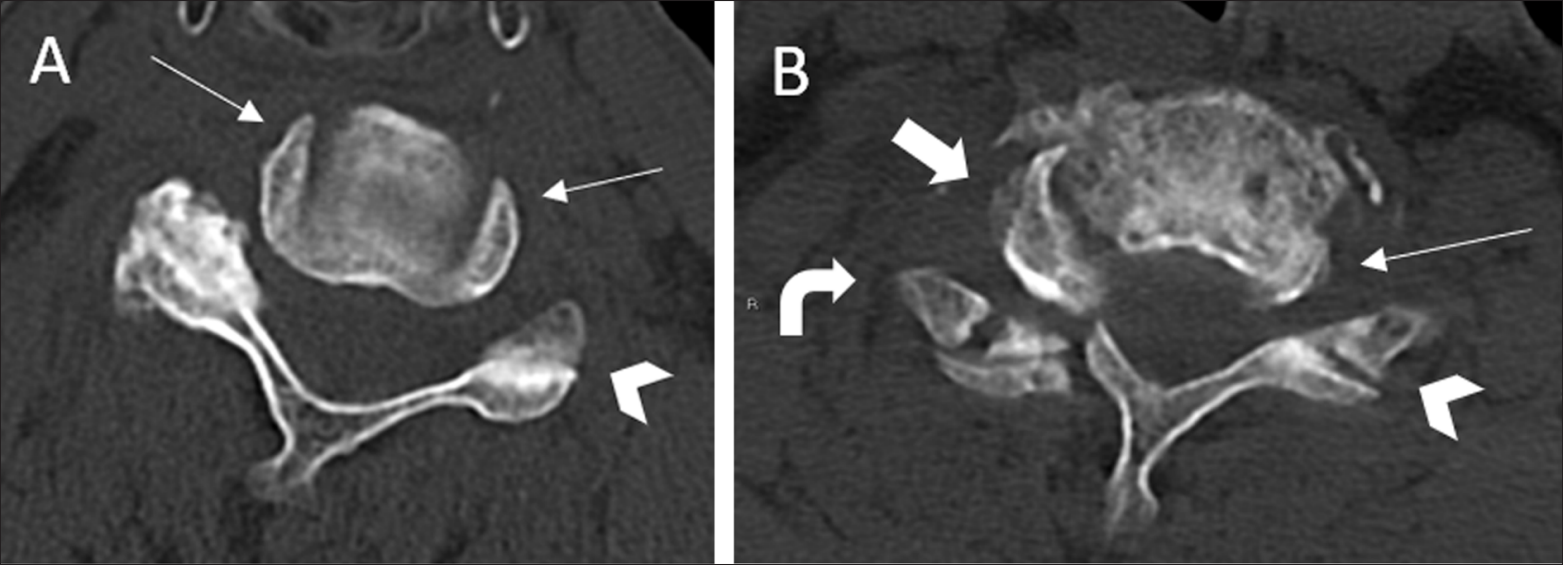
Headphones sign. Axial CT image of a cervical vertebra (A) shows normal concentric appearance of bilateral uncovertebral joints (arrows). This resembles the appearance of headphones on the wearer’s head. Axial CT image of the cervical vertebra (B) of an 80-year-old man following a fall accident shows a dislocated right uncovertebral joint (thick arrow). The vertebral body is no longer framed by the uncinate process of the lower vertebra; instead, it has moved anteriorly and is rotated towards the normal left side. This appearance gives rise to a unilateral positive “headphones sign” (thick arrow), which is commonly associated with unilateral cervical facet joint dislocation (UCFJD). In addition, the normal left facet joint resembles the appearance of hamburger buns (B, arrow head) while the dislocated right facet joint resembles a reverse hamburger bun (B, curved arrow), giving rise to the reverse “hamburger bun sign”. Hence, the positive “headphones sign” and reverse “hamburger bun sign” are both reliable indicators of cervical facet joint dislocation on axial CT imaging.
